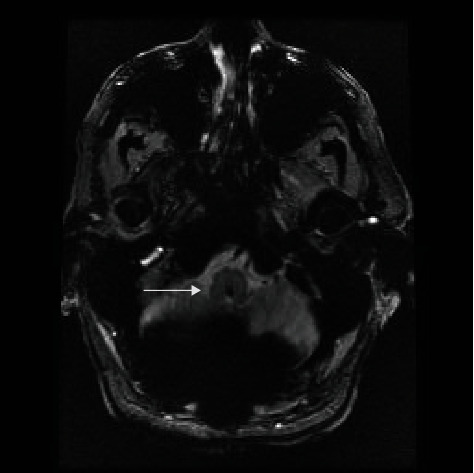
Postoperative cervical T2-weighted axial image demonstrates resolution of the caudal medullary cyst.
